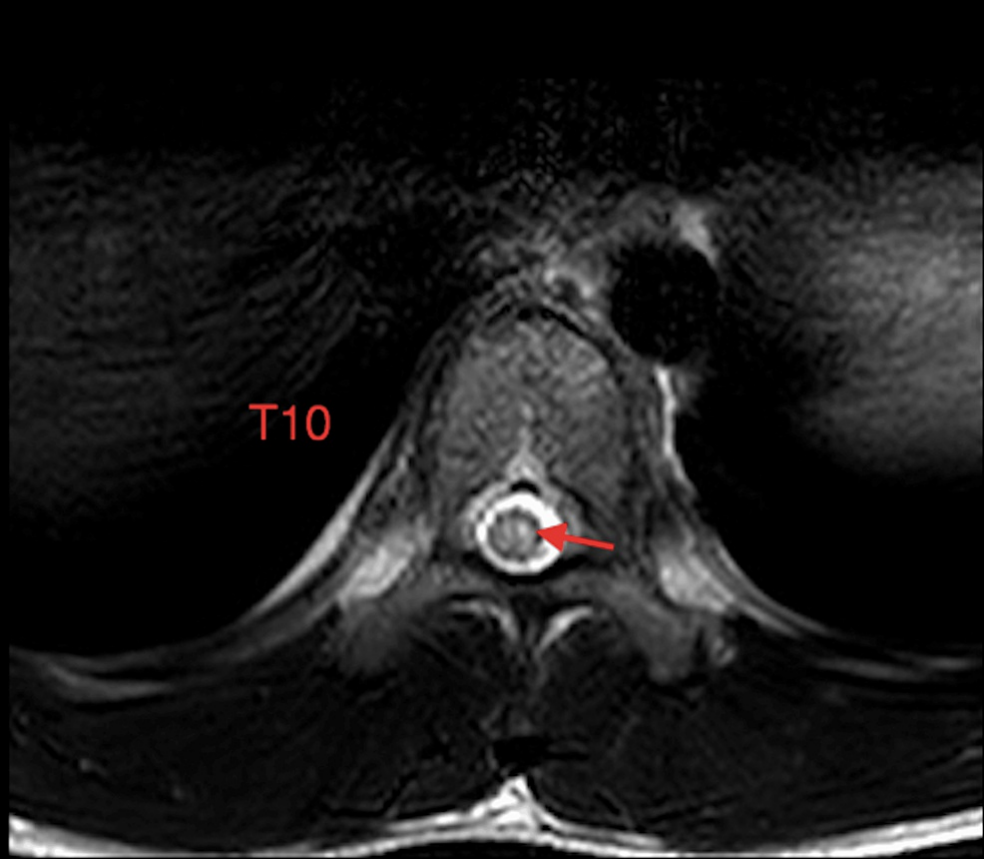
T2 weighted axial MRI image demonstrates high signal intensity in the central cord at the level of T10
